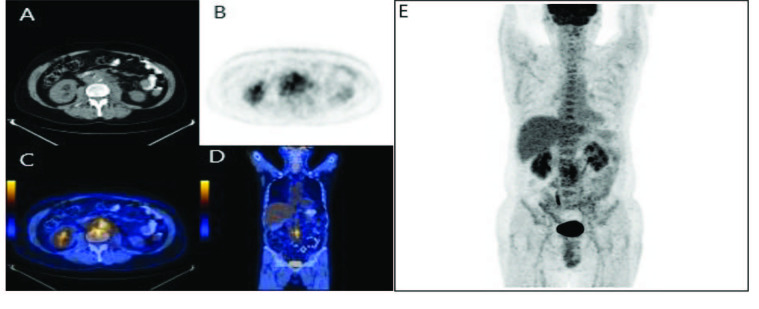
PET findings in RPF. 18F-FDG PET scans in a patient with idiopathic RPF show intense accumulation of ^18^F-FDG arround the abdominal aorta [A, axial CT, B, axial PET, C, axial fusion images, D, coronal fusion images, E, coronal maximum intensity projection (MİP) PET images)]. (Image courtesy of Özlem ATAY).
